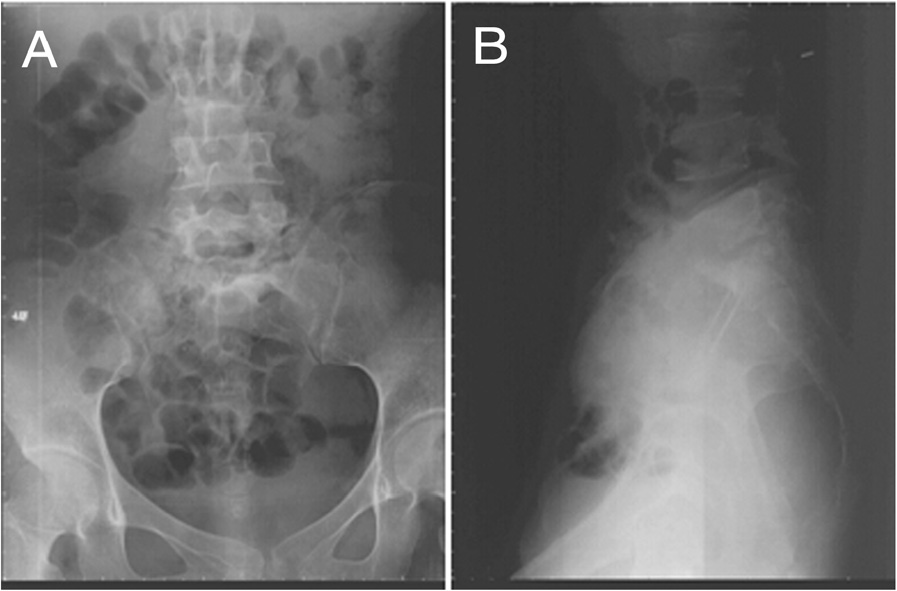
X-ray showing L5–S1 vertebral damage with narrowed intervertebral space and lumbosacral angle of 16°. (A) Anteroposterior view and (B) lateral view.
